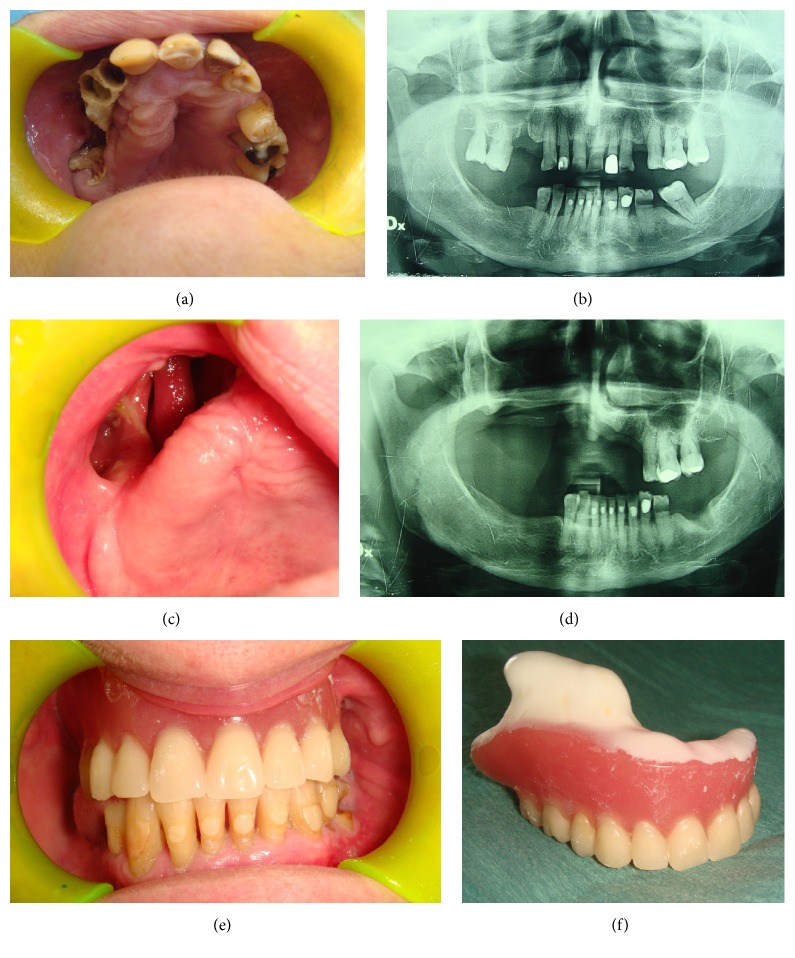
At clinical examination, presence of necrotic bone exposure (a) classified as stage III MRONJ depending on the dimensional staging system on the right maxilla in a female 67-year-old patient affected by breast cancer, who underwent denosumab administration 11 times. Rx OPT shows bone support reduction on the right maxilla. Patient referred the spontaneous loss of 4 teeth (1.7, 1.6, 1.3, and 1.2) after she underwent the orthopantomography, probably due to the progressive bone necrosis and resorption (b). After 16-month follow-up, clinical (c) and radiological (d) healing of the patient who underwent a removable prosthetic restoration (e-f).
